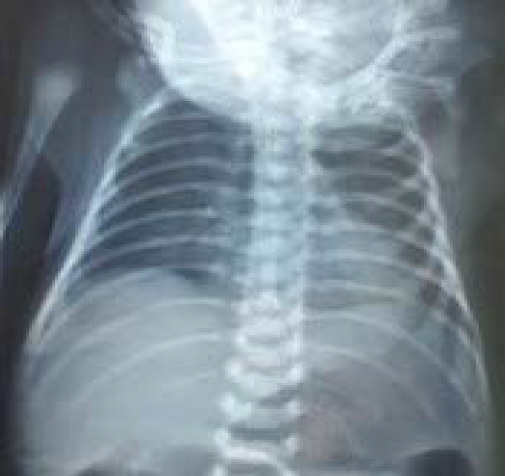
Repeat chest X-Ray showing resolved pneumothorax.
